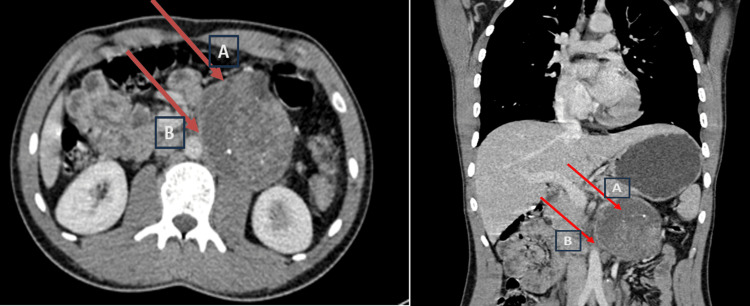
CT abdominal transverse section (left) and coronal section (right) The left retroperitoneal tissue mass contains calcifications and necrotic areas (red arrow A) and has intimate contact with adjacent vascular structures, including the left vein (red arrow B).
